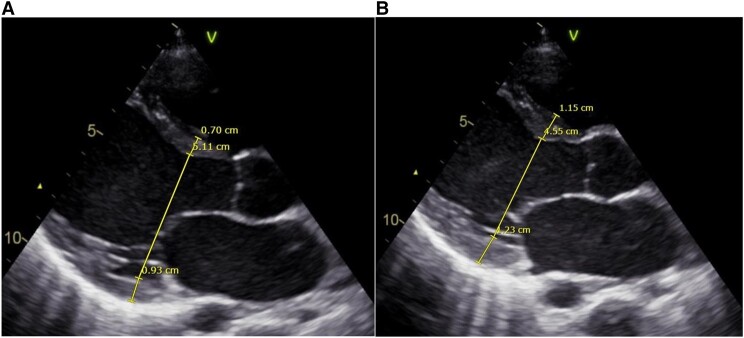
PLAX view of end-diastolic (A) and end-systolic (B) echocardiographic image at presentation.
